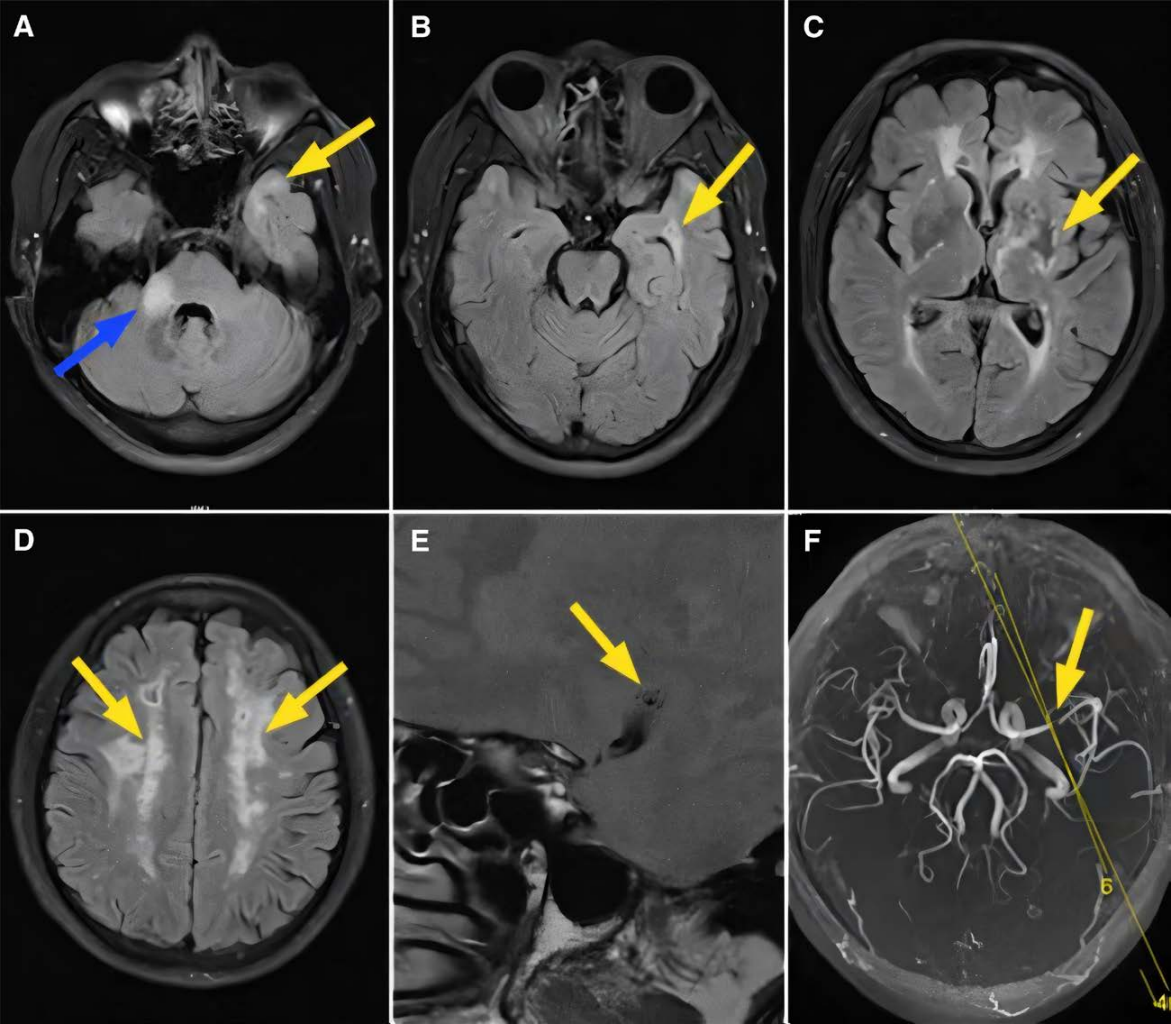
Brain magnetic resonance imaging of the proband. (A) FLAIR image showed acute infarction in the right cerebellar root (Blue arrow), and white matter lesion in the temporal region (yellow arrow). (B and C) FLAIR image showed temporal white matter lesions and external capsule lesions (yellow arrow). (D) FLAIR image showed extensive fused white matter high signal in the bilateral hemianopia (yellow arrow). (E and F) High-resolution MR + MRA of the brain revealed severe stenosis of the left middle cerebral artery, and the inferior plaque of the stenosis was strengthened, yellow line is E positioning line.
